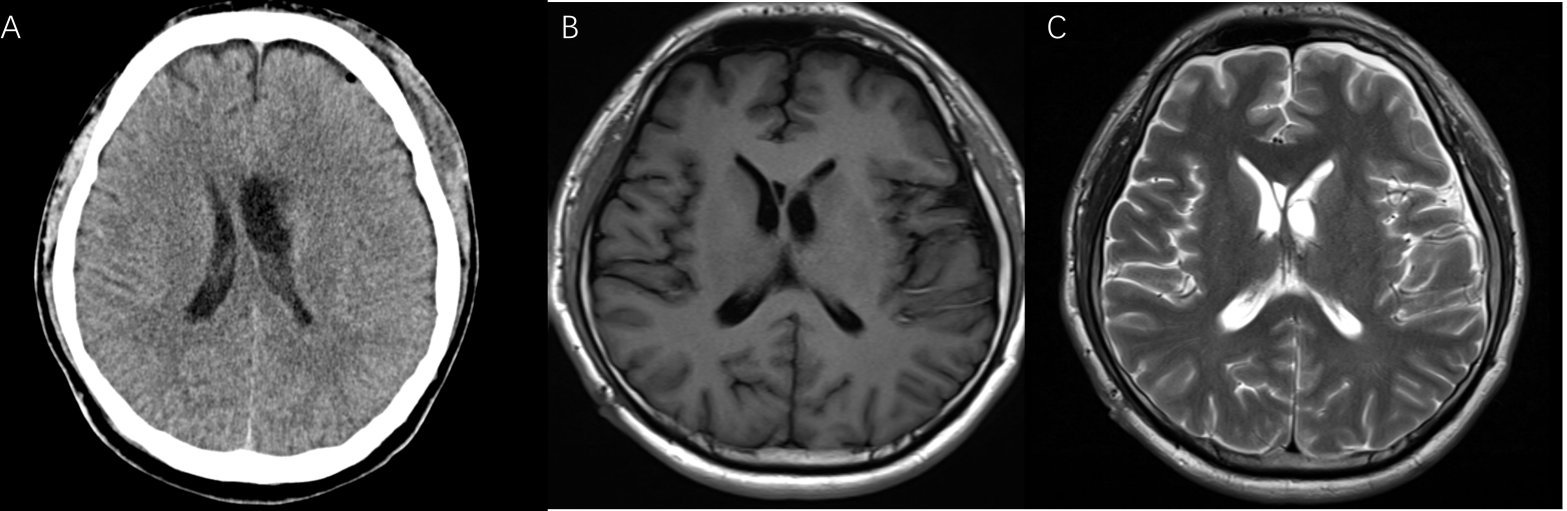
The postoperative CT (A), T1-weighted (B), and T2-weighted MRI (C) after one month showed good tumor resection.
